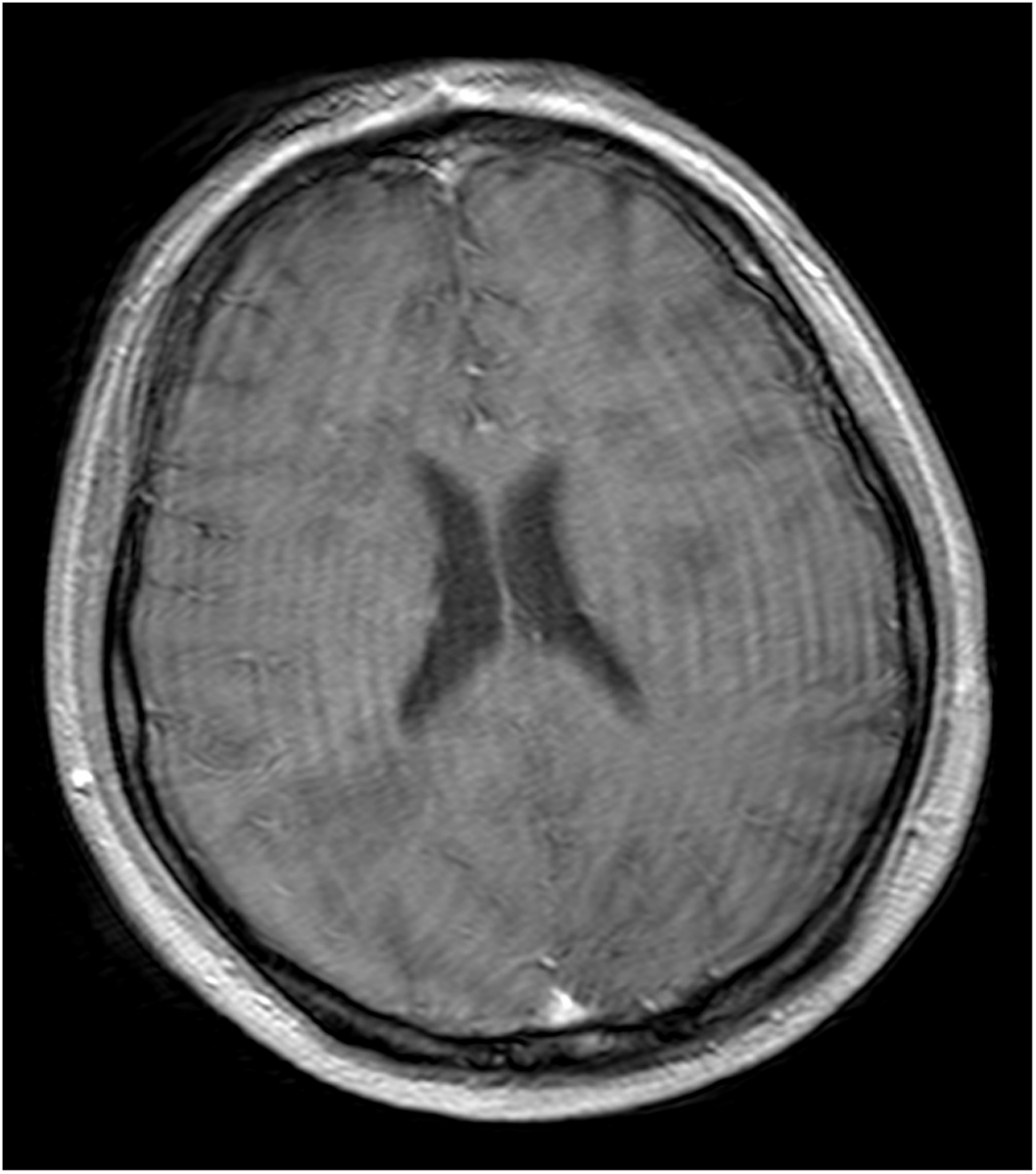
Magnetic resonance imaging with contrast done 5 days post‐treatment with intravenous immunoglobulin, IV methylprednisolone, and rituximab. Showing that the post‐contrast T1 enhancement in the nodular lesions resolved.
